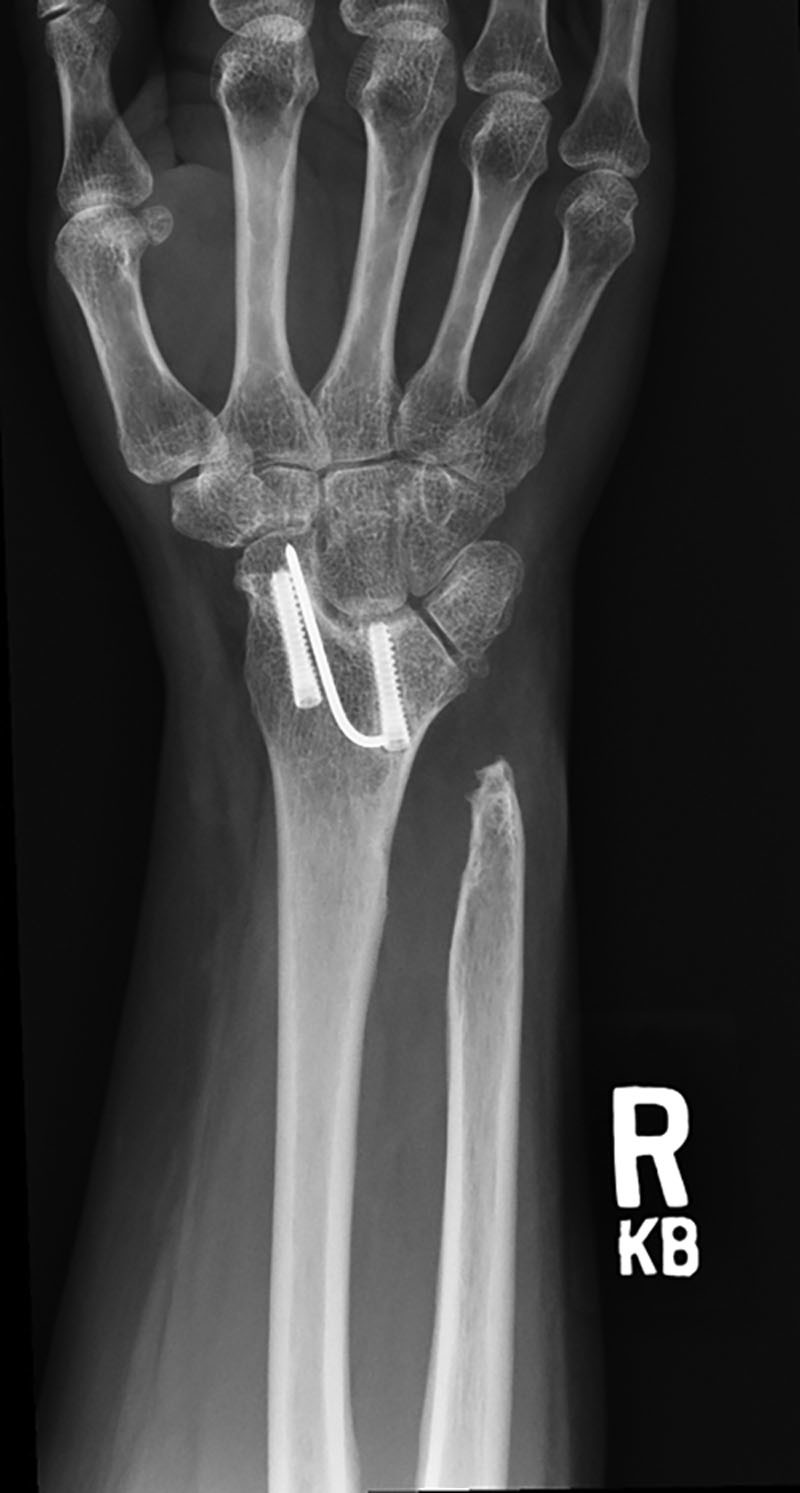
Patient 1 9-month postoperative roentgenogram showing no recurrence of heterotopic ossification.
